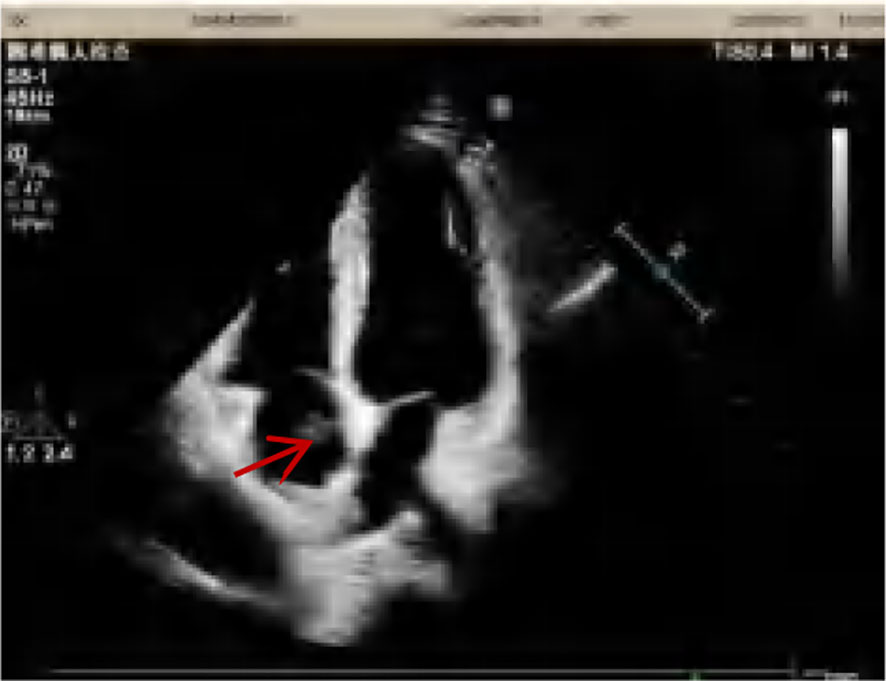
Preoperative cardiac ultrasound, the arrow points to a cancer embolus.
